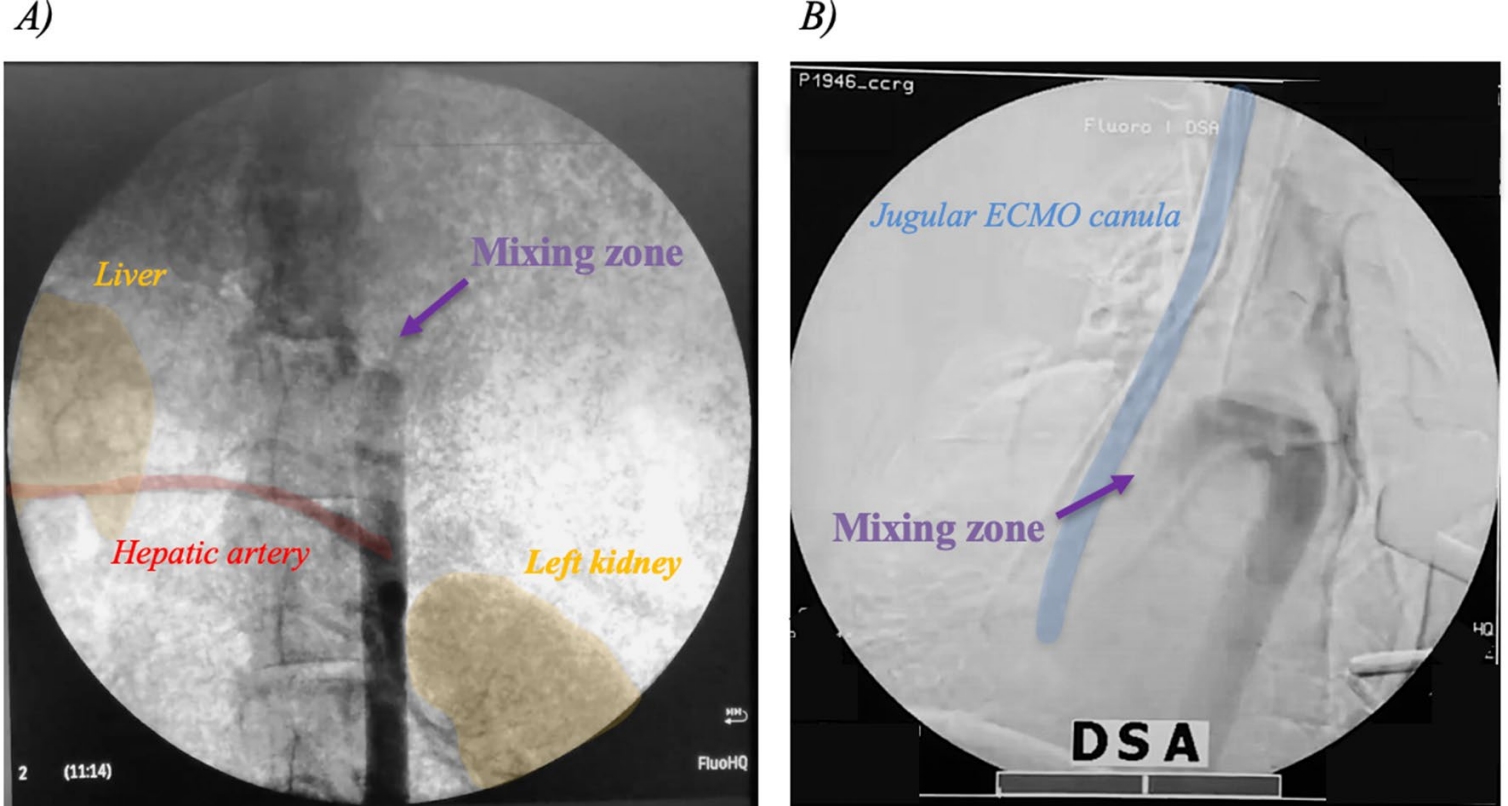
X-ray images of the mixing zone. Legend: The mixing zone corresponds to the zone where the LV and the ECMO meet. (A) Low flow: the mixing zone is in the abdominal aorta above the hepatic artery. (B) High flow: the mixing zone is in the ascending aorta (the whole aortic arch is opacified). ECMO = extracorporeal membrane oxygenation; LV = left ventricle.
